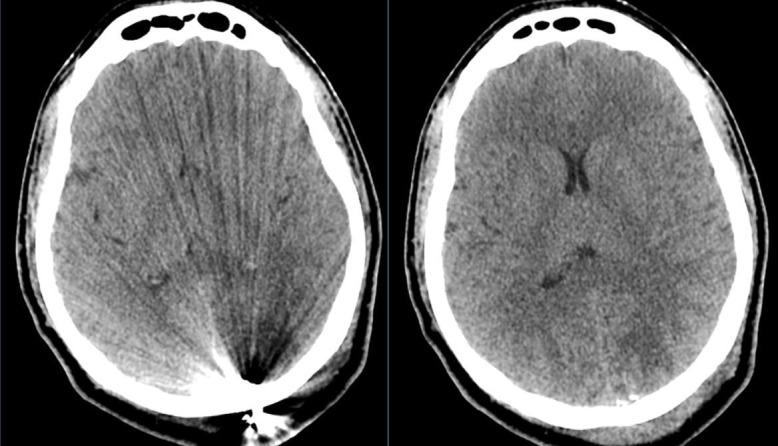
Computed tomography (CT) head revealed a comminuted, depressed left skull fracture, a retained high-velocity projectile in the midline occipital region, and minimal pneumocephalus.
